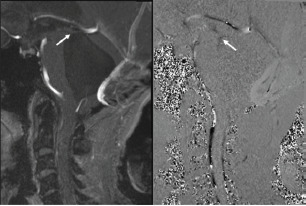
Magnetic resonance imaging brain cine protocol demonstrating obstructed flow near the exit point of the cerebral aqueduct into the 4th ventricle (white arrow).
